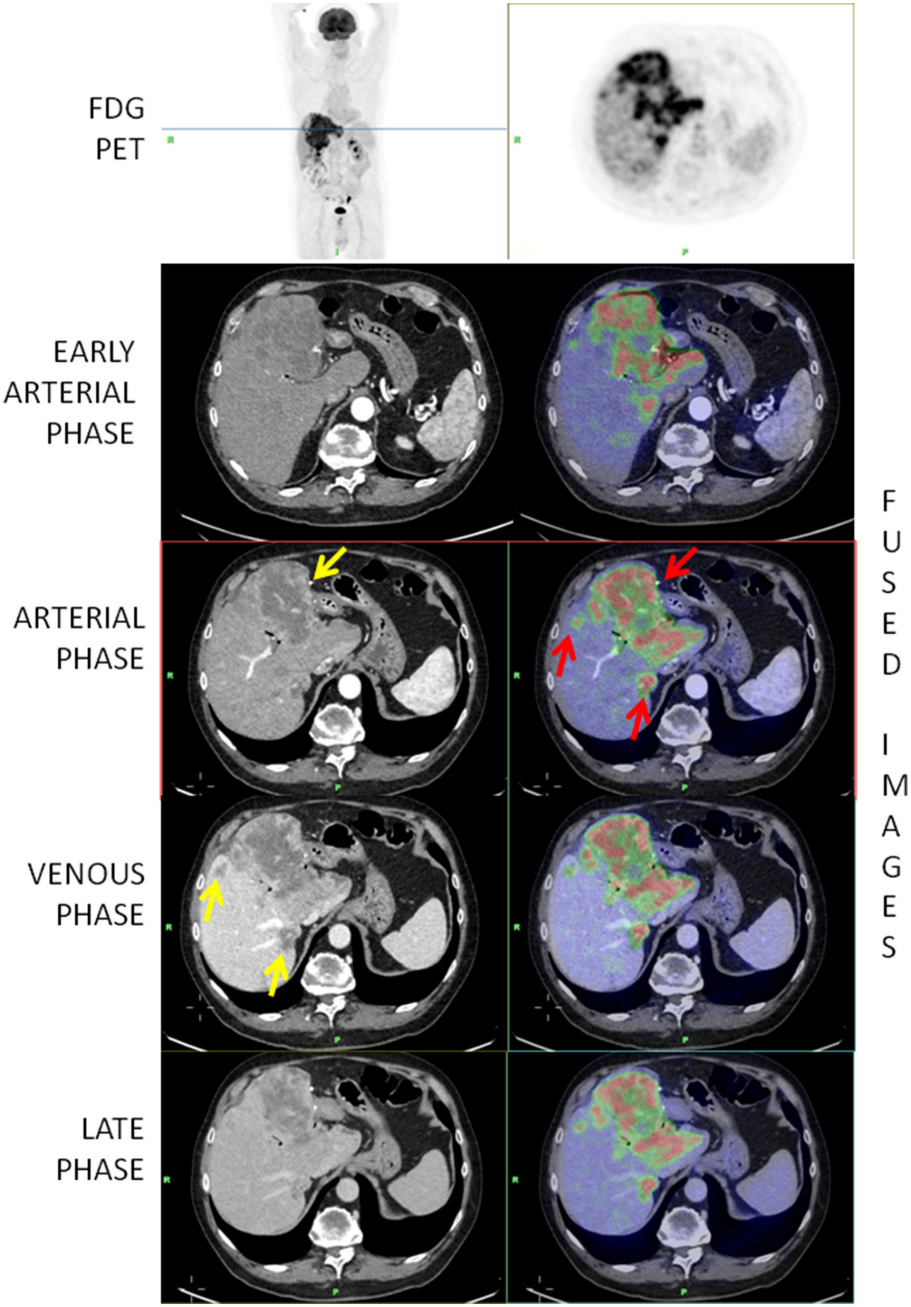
[^18^F]-FDG-PET/ceCT showing a highly metabolic primary iCCA and two hepatic secondary lesions (red arrows). The yellow arrow on the arterial phase shows a peripheral vascularization while the arrows on the venous phase show that secondary lesions are better depicted in the venous phase.
